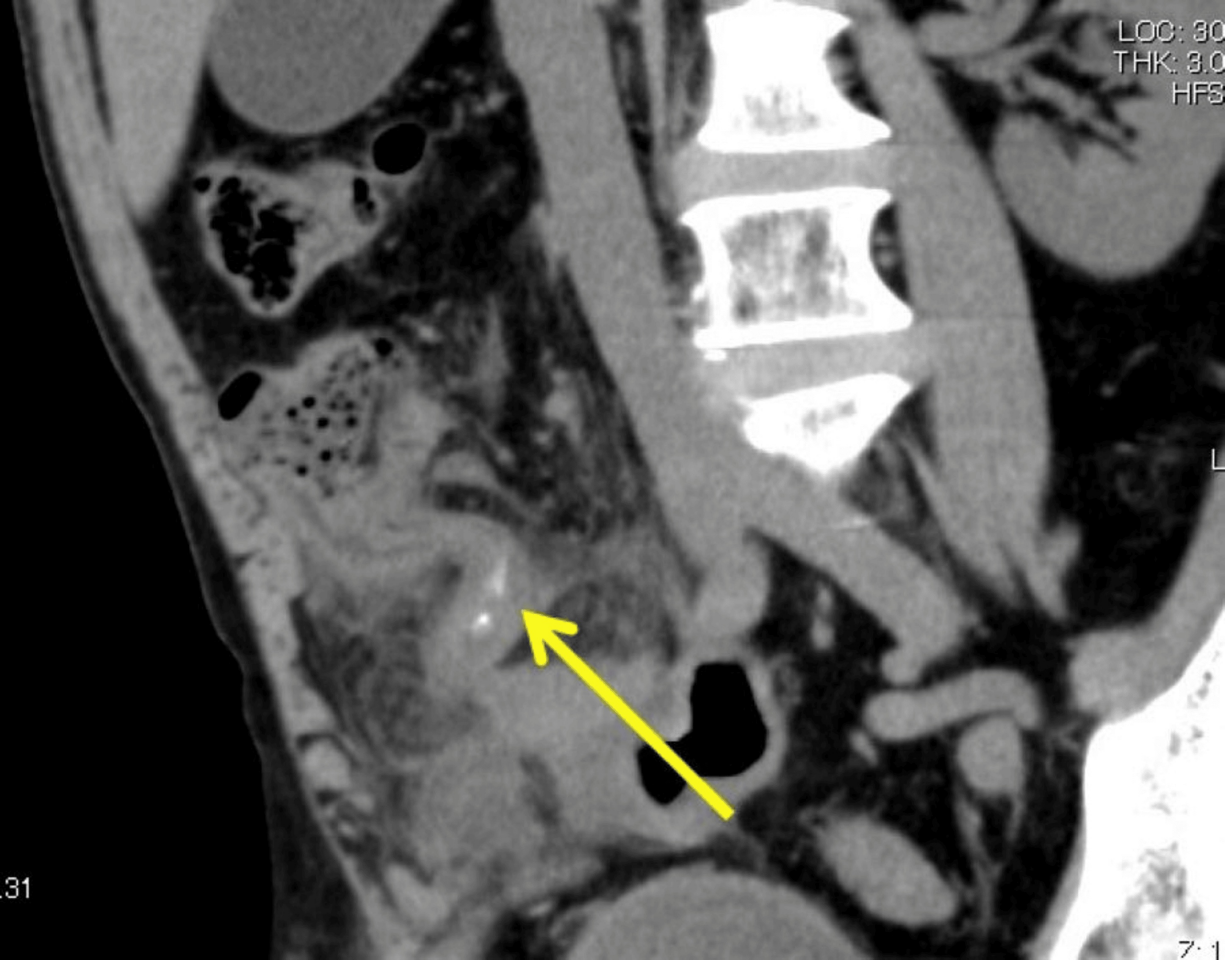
The multiplanar reconstruction (MPR) image from the abdominal CT scan. Postoperative MPR images reconstructed along the longitudinal axis of the appendix revealed a wedge-shaped hyperdense linear structure (yellow arrow) within the appendix, which was identified as a fish bone. The tip of the fish bone appeared to penetrate the appendiceal wall.
